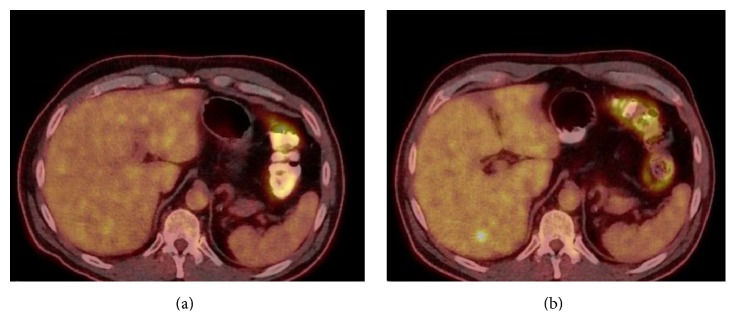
PET/CT at 4 months (a) and 8 months (b) after total thyroidectomy. There is new focal uptake within the posterior right lobe of the liver, measuring a maximum SUV of 5.4, consistent with metastatic disease. This was not evident on the initial PET/CT.
